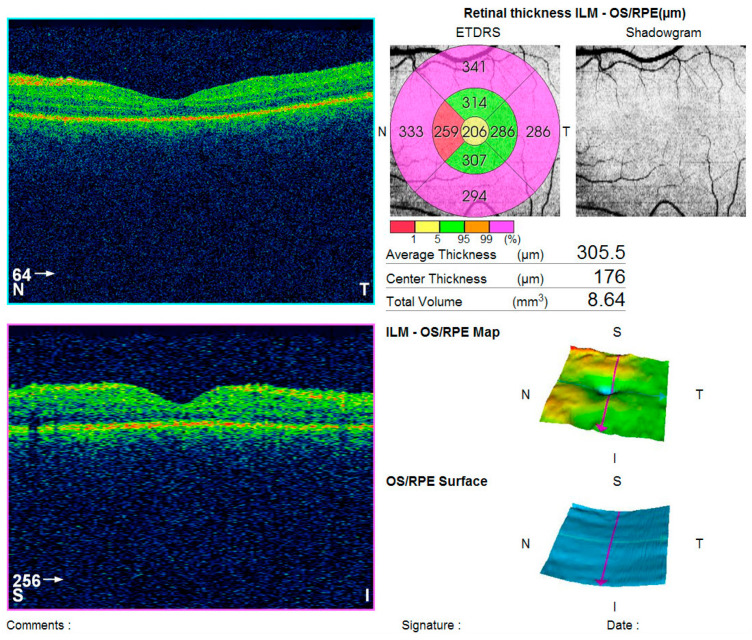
CT (Topcon 3D OCT-2000) of the left macula showing edoema resolution.
